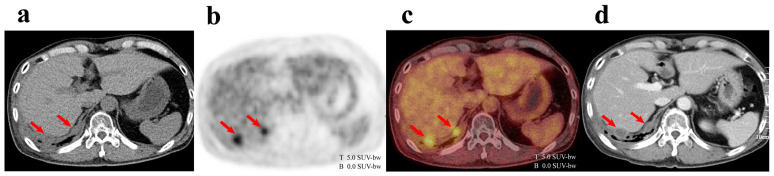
Local recurrence detected on ^18^F-fluorodeoxyglucose (FDG) positron emission tomography/computed tomography (PET/CT). A 49-year-old man underwent pleurectomy/decortication for malignant pleural mesothelioma. FDG PET/CT performed at 10 months postoperatively (a–c) demonstrated focal FDG uptake in the postsurgical bed of the right lower hemithorax, with focal pleural thickening on CT (arrows), consistent with local recurrence. Follow-up chest CT obtained 3 months later confirmed progressive nodular pleural thickening (d). Spatial scale bars (10 cm) and FDG uptake intensity scales (0.0–5.0 SUV-bw) are displayed for reference. T and B indicate the top (maximum, 5.0 SUV-bw) and bottom (minimum, 0.0 SUV-bw) of the FDG uptake intensity scale, respectively.
